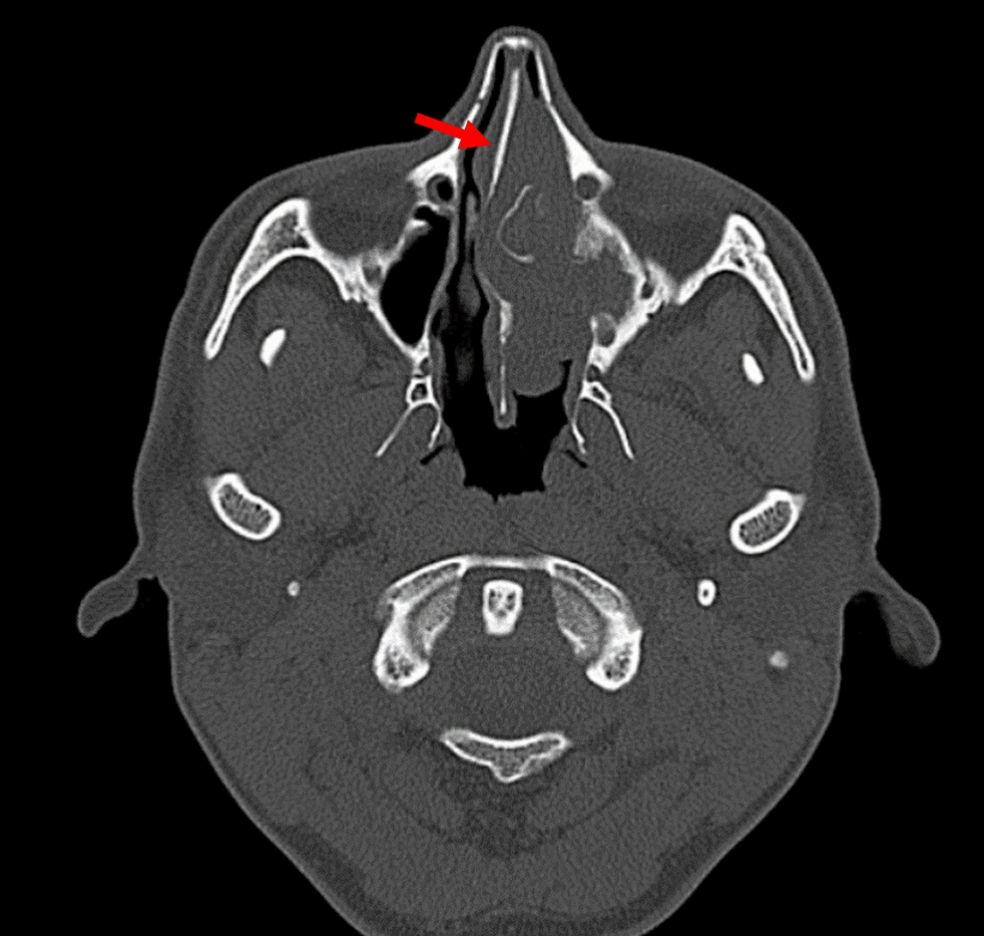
CT scan (bony window) axial cut shows soft tissue lesion filling the left nasal cavity completely with rightward deflection of the nasal septum. Rightward deflection of the nasal septum (red arrow).
